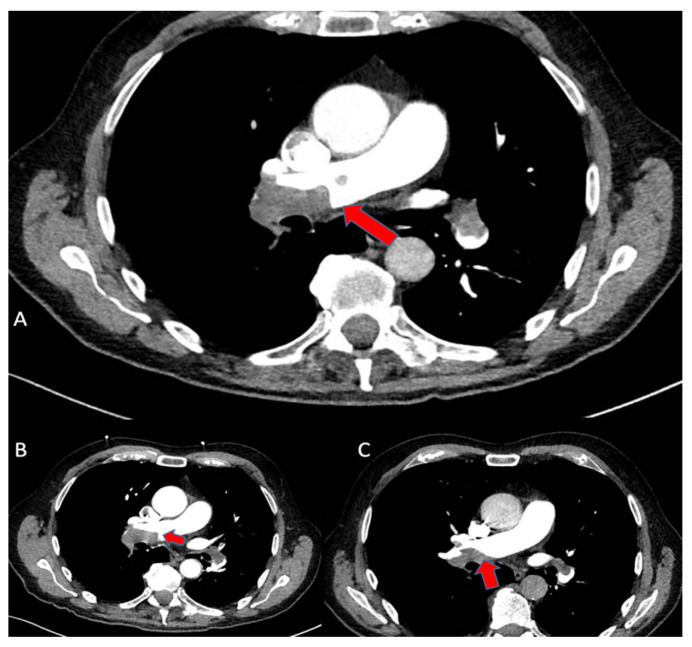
(A): CT scan at baseline showing PE; (B): CT scan performed after EKOS treatment showing very limited reduction in emboli; (C): CT scan showing a significant reduction in thrombotic burden after thromboaspiration with FlowTriever. The red arrows show the thrhombotic formations in the right pulmonary artery.
